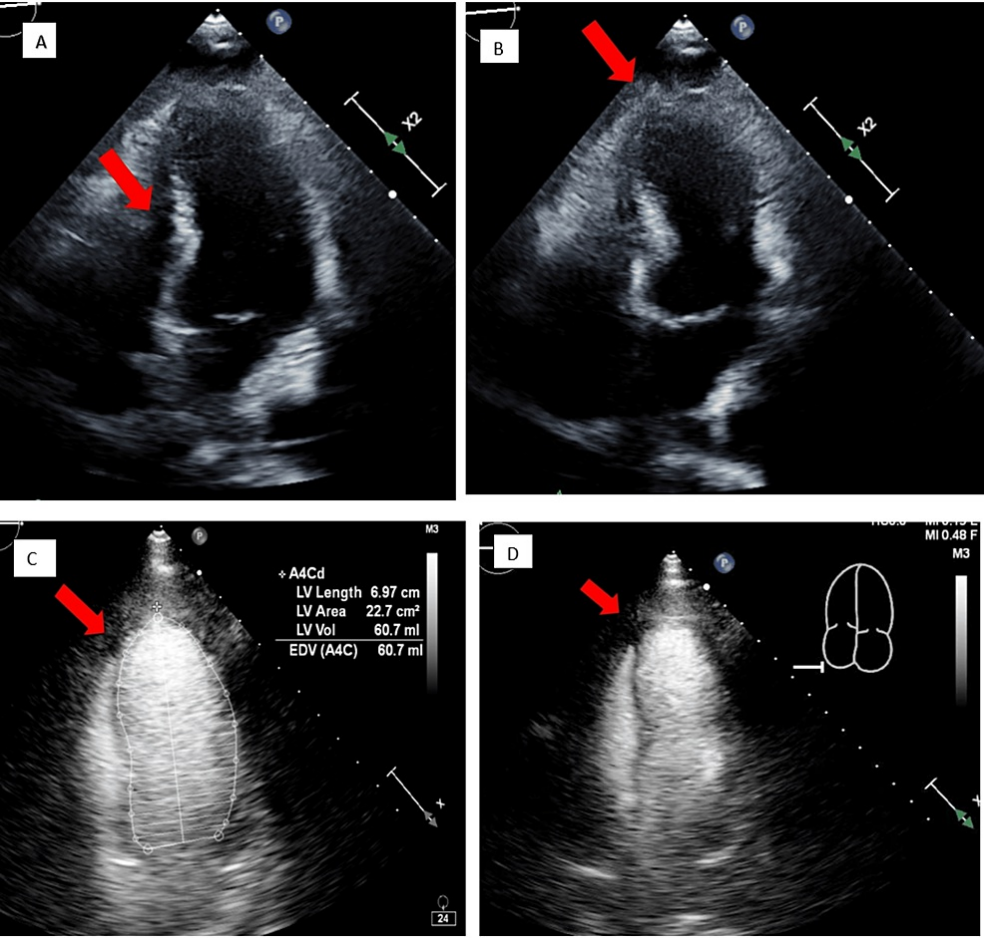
Echocardiogram images of hypokinetic mid-apical segments with preserved contractility of basal segments (Takotsubo cardiomyopathy) during systole and diastole. Figures A and B are without contrast, and Figures C and D are with contrast images.
